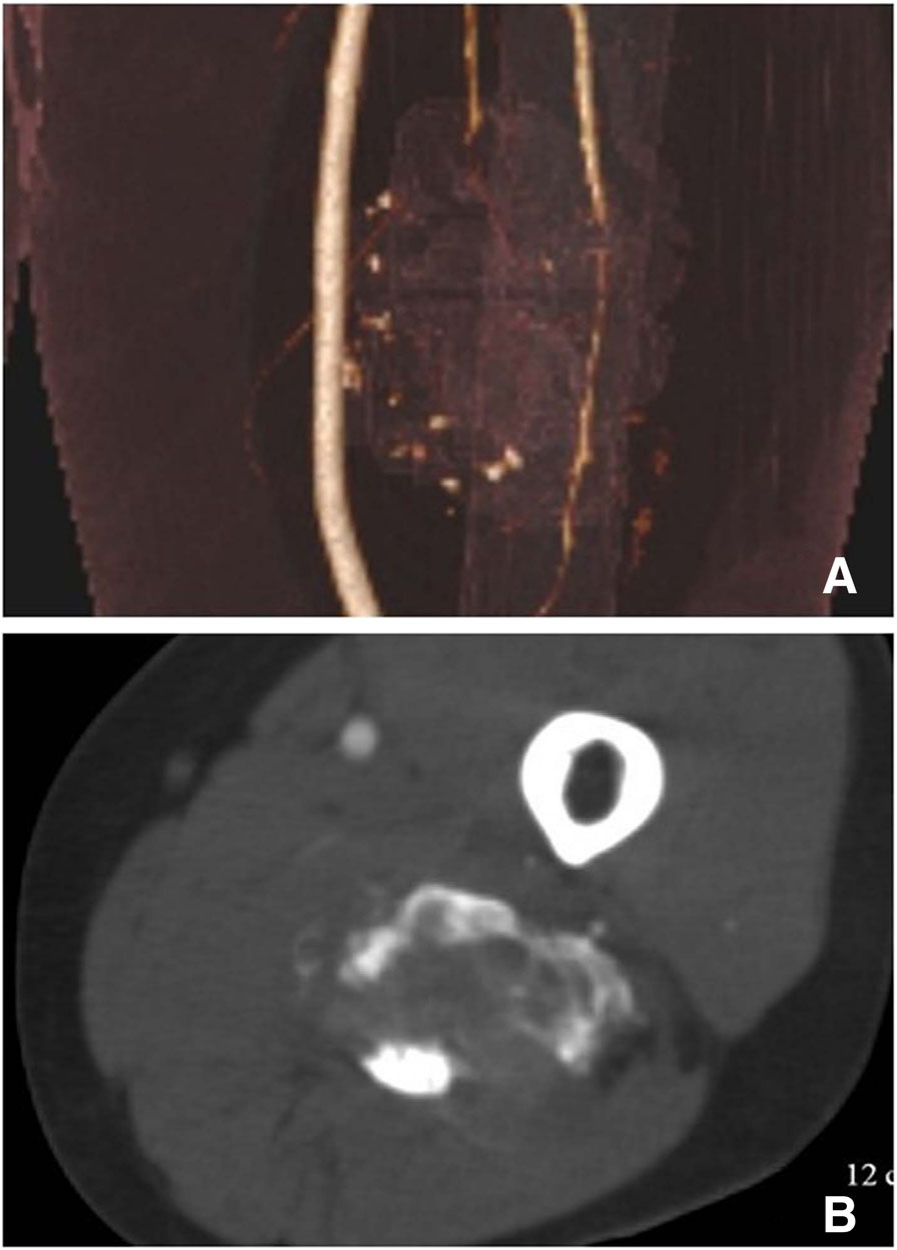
LGMS of the left thigh. CT scan revealed an irregular mass (a) with calcification inside (b)
